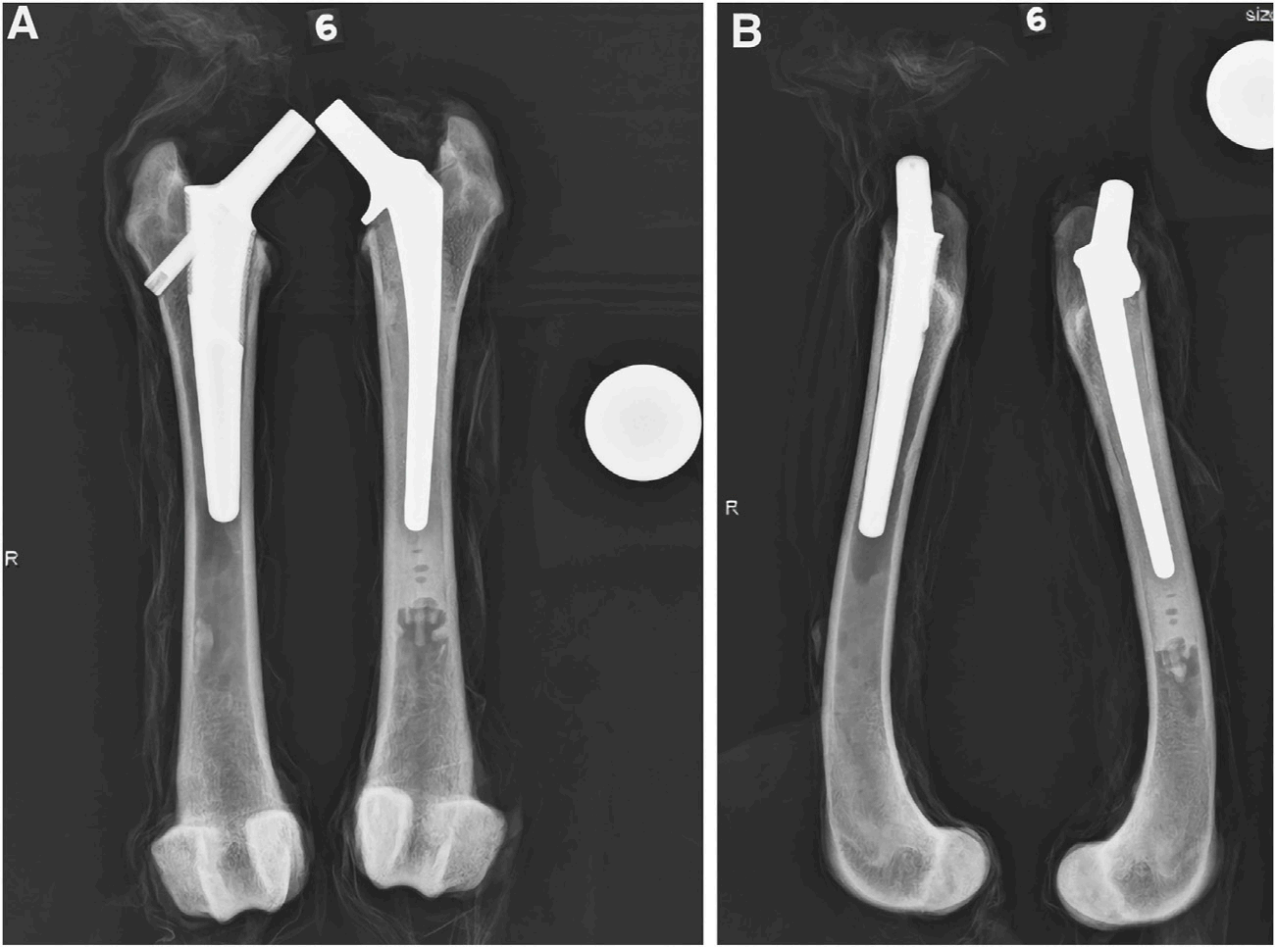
Representative cranial-caudal (A) and medial-lateral (B) radiographic views after stem implantation. The BFX + lb implanted femur is on the left and CFX implanted femur is on the right.
